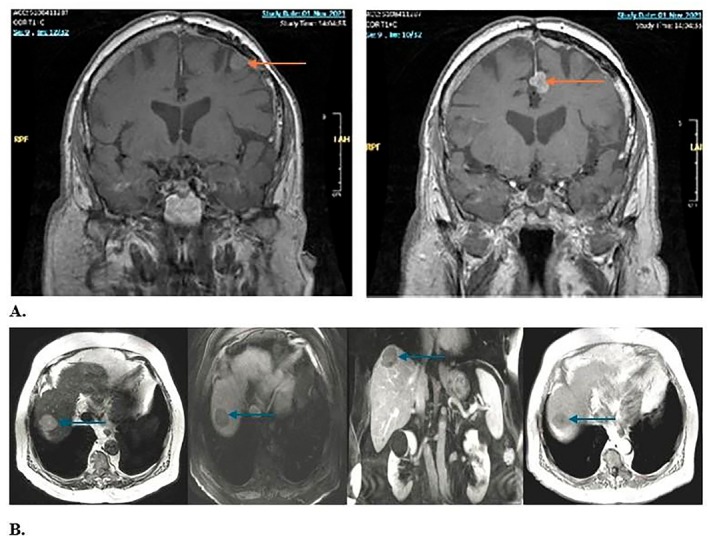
A. Brain MRI showing multiple meningiomatous lesions, November 2021. B. Abdominal MRI showing the hepatic metastasis of the meningioma.
